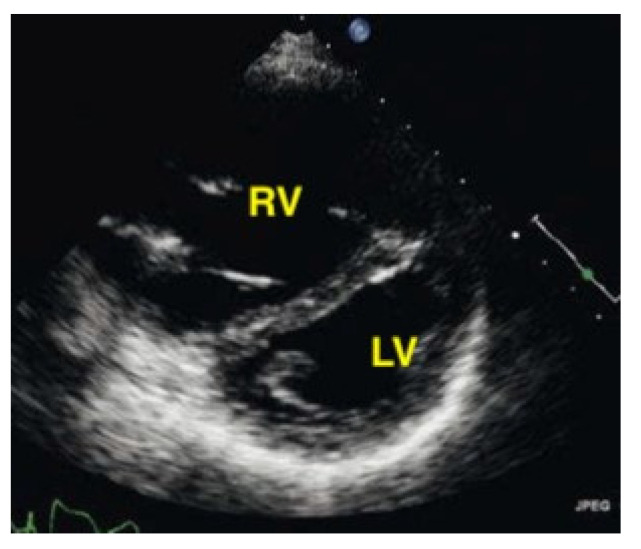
Parasternal short-axis view. The pathologically increased right ventricular pressure pushes the septum to the left side during diastole, so that a D-shape of the left ventricle results (RV = right ventricle, LV = left ventricle).
